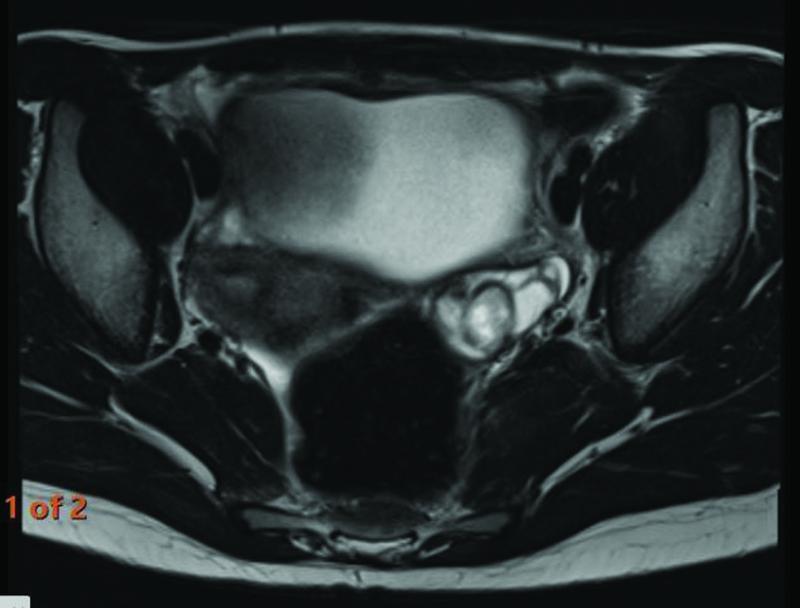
Magnetic resonance imaging (MRI) demonstrating an axial view of the metachronous left ovarian teratoma preoperatively.
